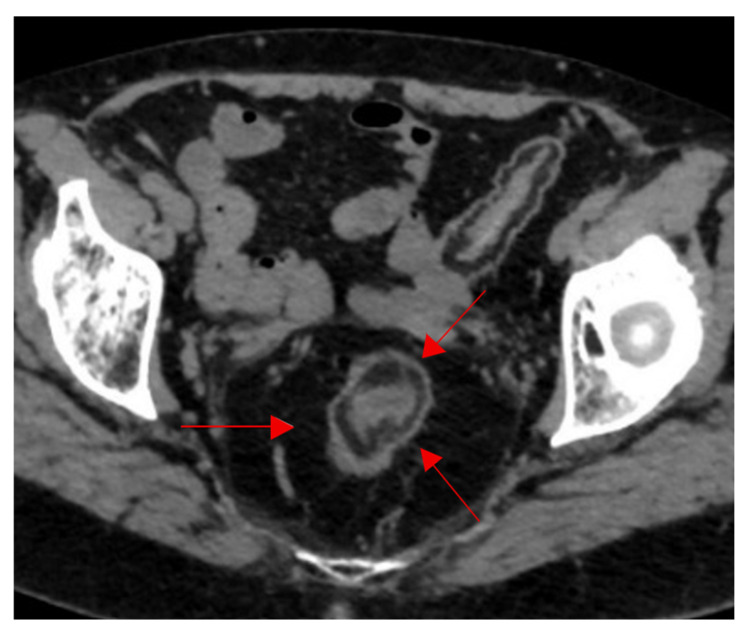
Fat halo sign in longstanding ulcerative colitis. Infiltration of the submucosa with fat between the muscularis propria and the mucosa at the level of the sigmoid colon on axial CT images of the pelvis (red arrows).
